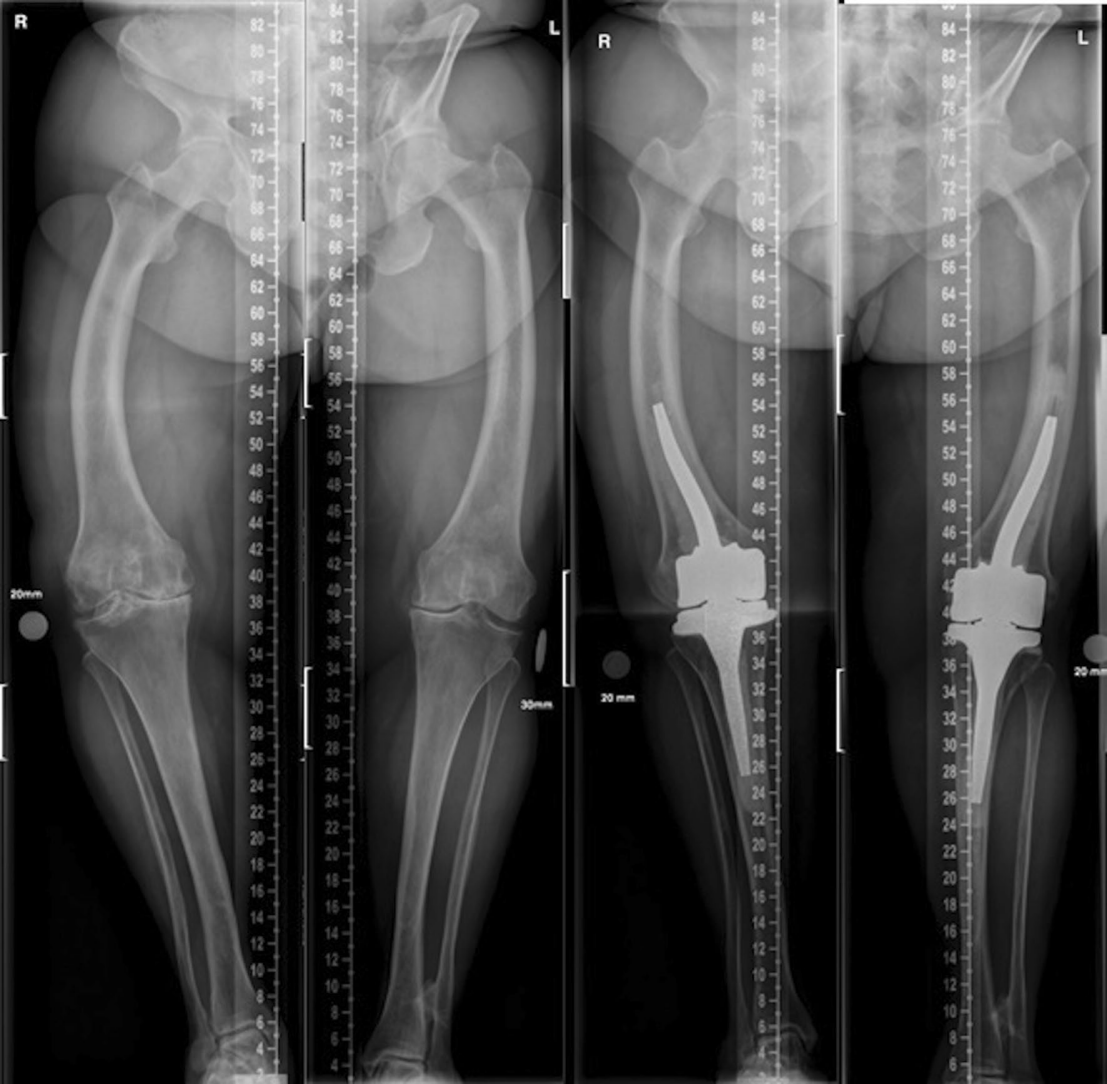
Familial hypophosphatemia patient. Pre- and post-operative long leg weight-bearing radiographs of a patient with familial hypophosphatemia. Pre-operatively, osteotomy and TKA were considered, but, due to an increased risk of complications (diabetes, smoker), the surgeon decided to first perform CMH on the right knee. The patient was pleased with the outcome, and the left knee was also operated upon five months later using the same technique. The post-operative MA was 9 (right) and 2 (left) degrees of varus
